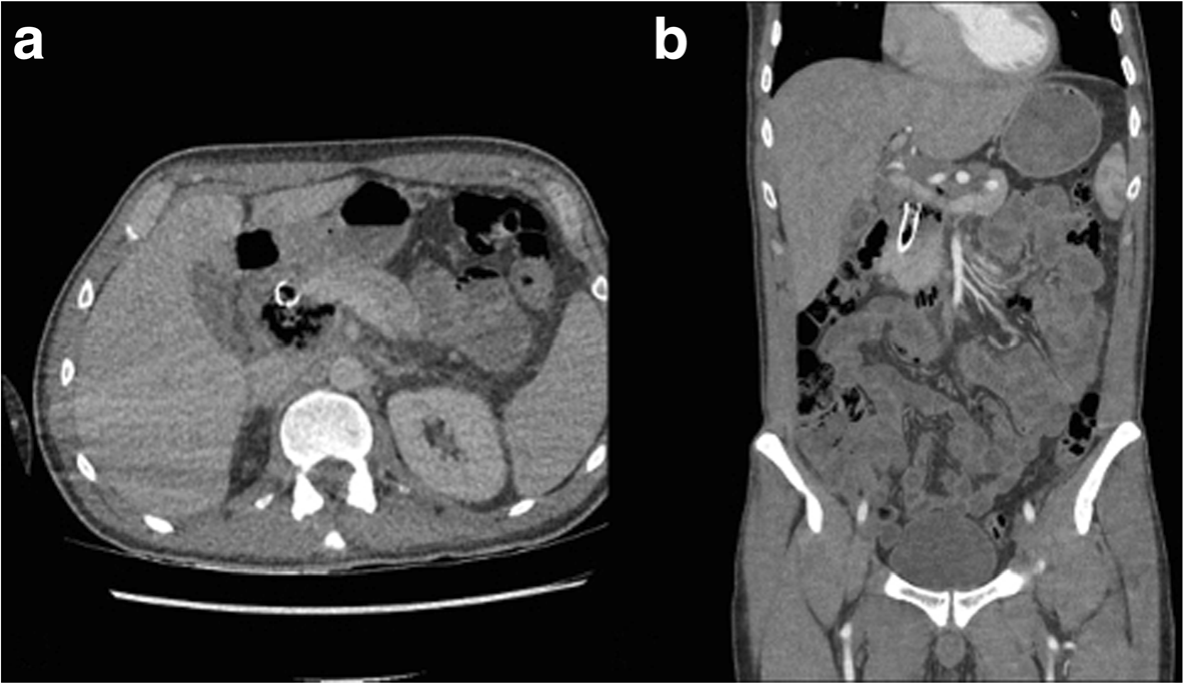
Abdominal CT following one dose of chemotherapy showing necrosis of the periportal nodal mass and erosion of the stent through the bile duct
